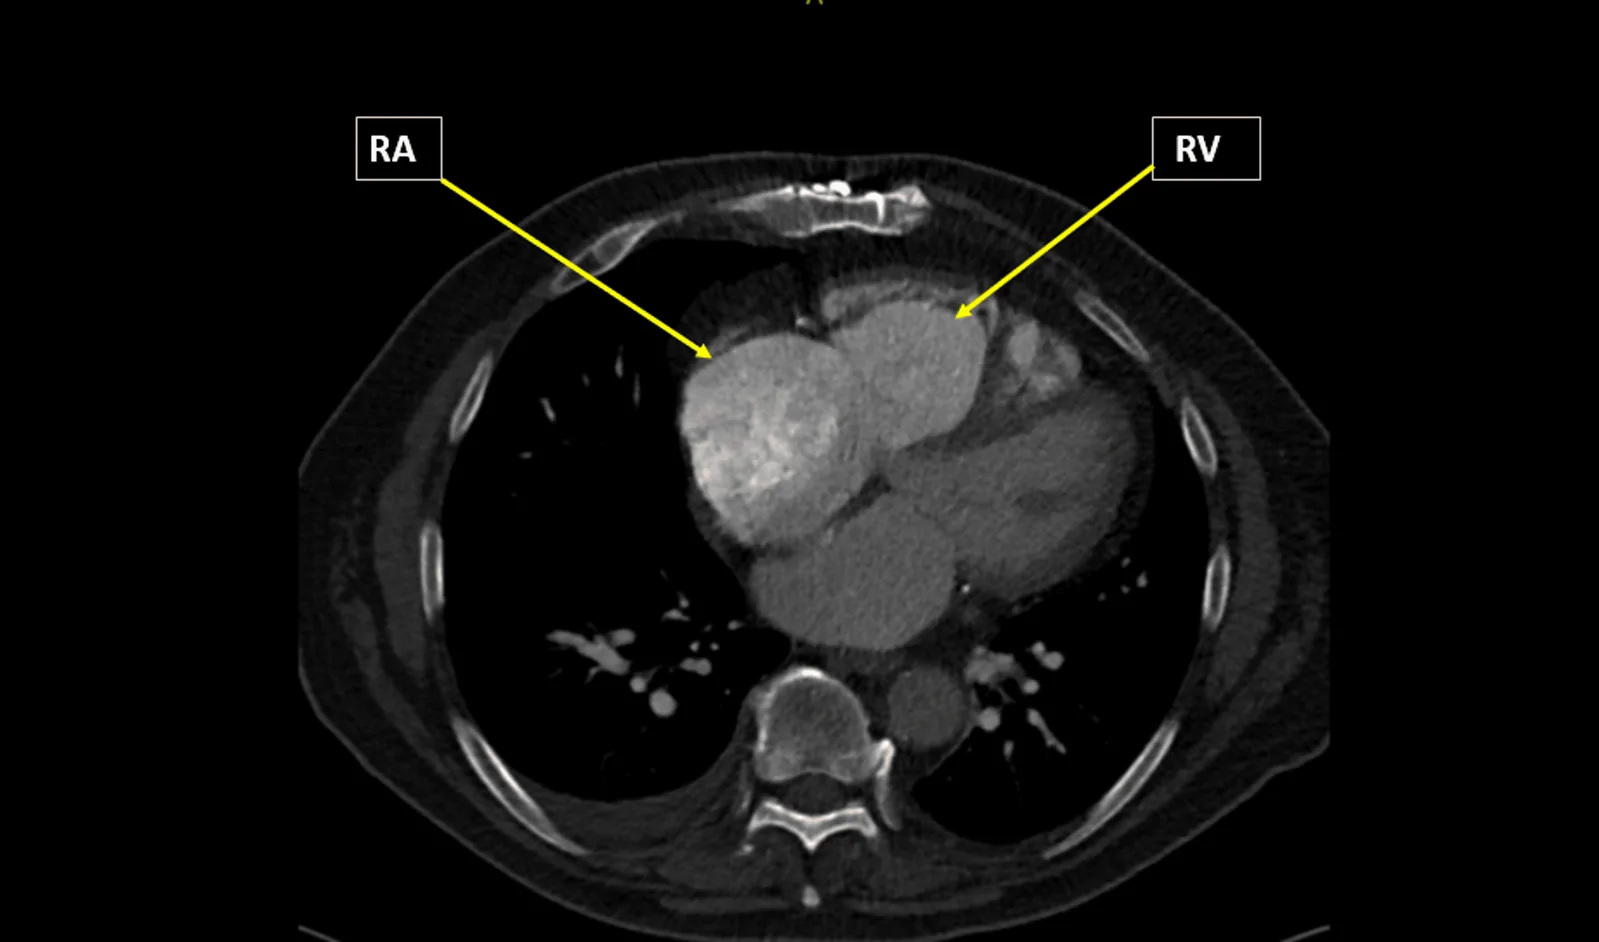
Contrast-enhanced CT chest demonstrating right-sided cardiac chamber enlargement Axial contrast-enhanced CT demonstrating right atrial (RA) and right ventricular (RV) enlargement, consistent with chronic right ventricular pressure overload secondary to pulmonary hypertension.
